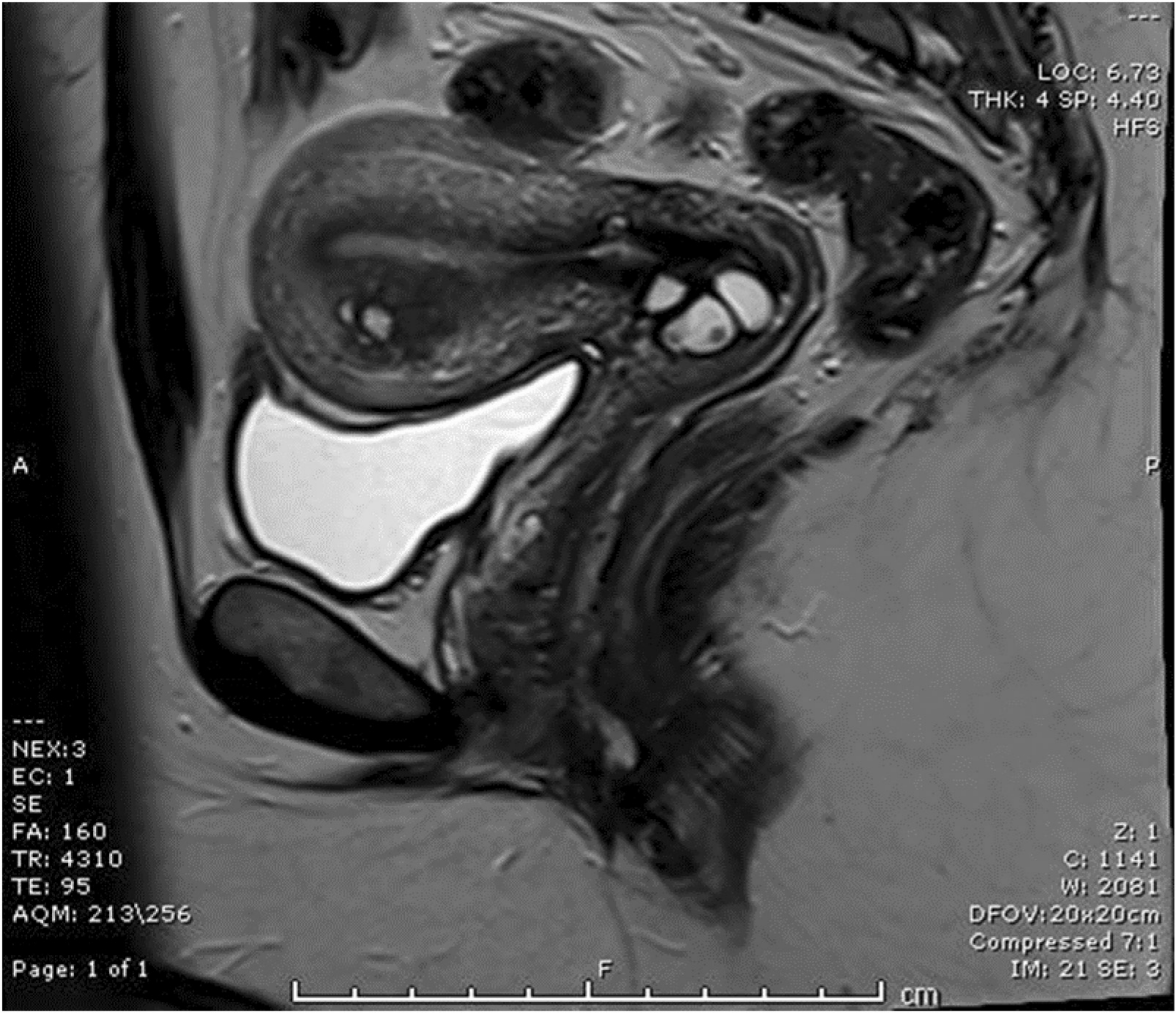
MRI scan showing fundal cystic structure
